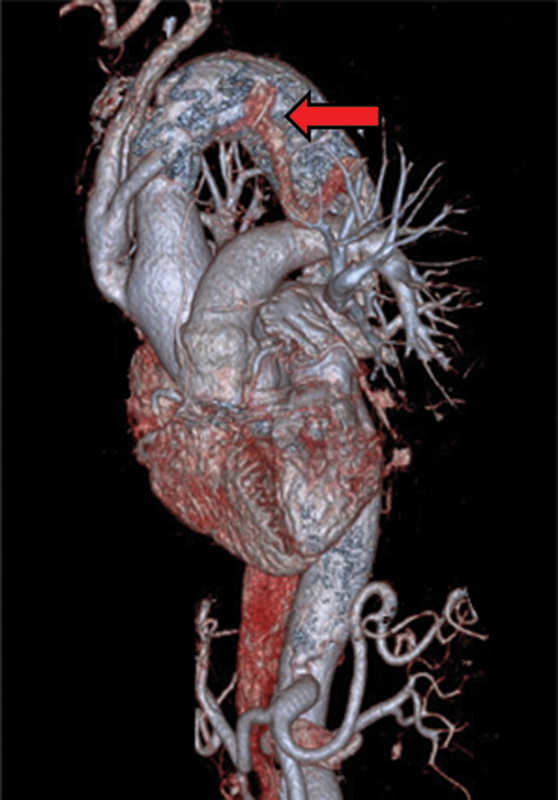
Reformatted postoperative three-dimensional computed tomography scan showing the debranched aorta and endovascular stent graft (red arrow).
